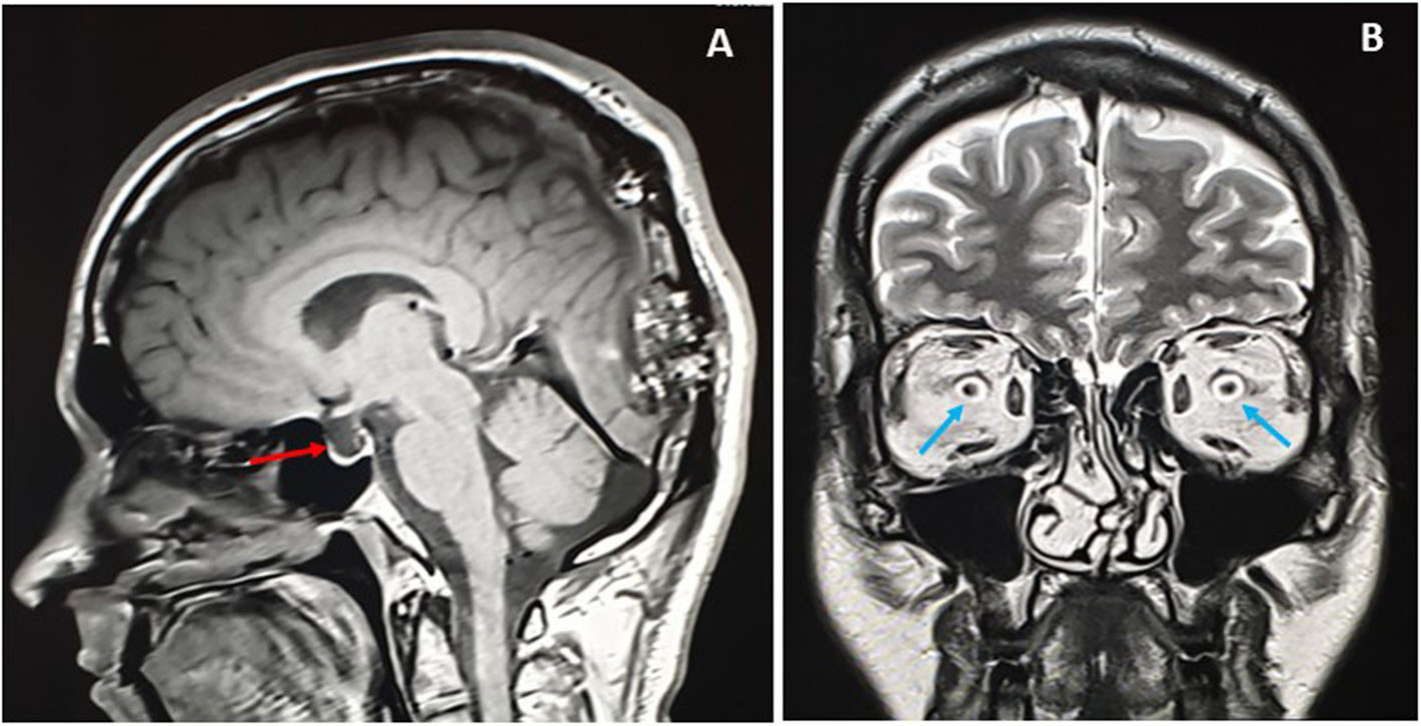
Sagittal T1 MRI image showing empty Sella turcica (red arrow) (a) and distended optic nerve sheaths (Blue arrows) on coronal T2 (b)
